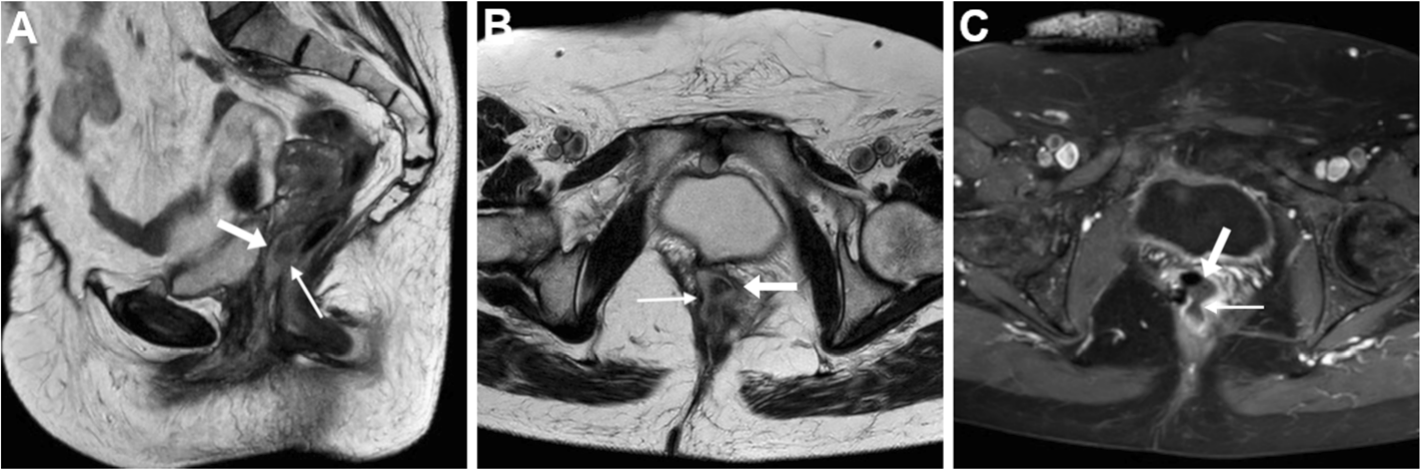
Recto-VF after surgical treatment of bladder and uterine prolapse with prosthetic mesh placement. T2-weighted (a, b) and FS post-gadolinium T1-weighted (c) MRI images show wide fluid-filled communication (thin arrows) between the distal rectum and upper third of the vagina (arrows), with marked peripheral enhancement in (c). Note poor visualisation of the mesh (few artefacts abutting the right side of the VF on c)
